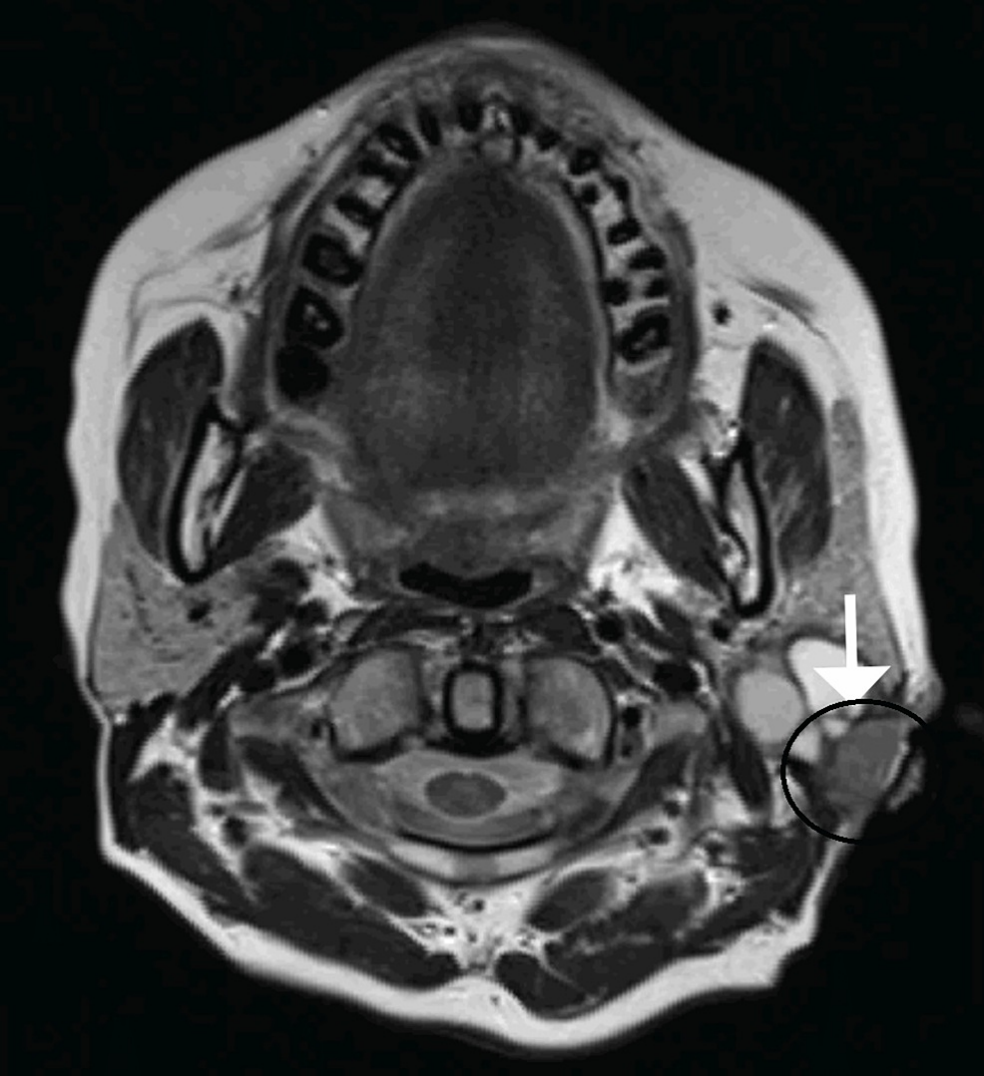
MRI image showing a tumor in the left parotid gland involving the fasciovenous plane of Patey
